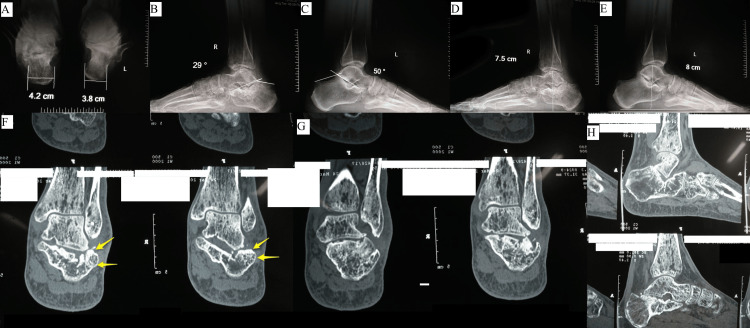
(A-E) Lateral and Harris axial views of the injured and normal calcaneus showing loss of TCH and BA and widening of the heel. (F, G) Coronal CT cuts depicting lateral wall blowout and subfibular impingement (yellow arrows). (H) Sagittal CT sections demonstrating arthritic changes in the subtalar joint BA, Böhler’s angle; TCH, talocalcaneal height
